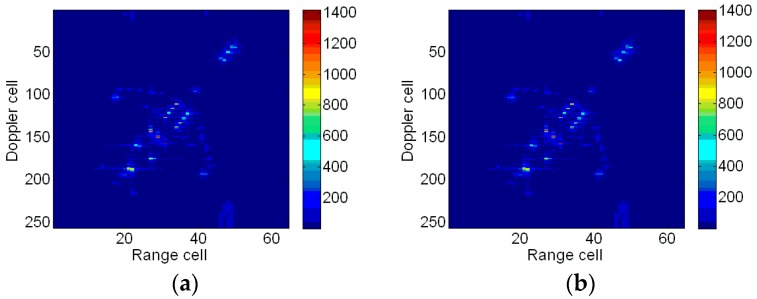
ISAR imaging results. (a) ISAR image with optimal relative chirp rate; and (b) ISAR image with an optimal relative chirp rate and Doppler focus shift.
